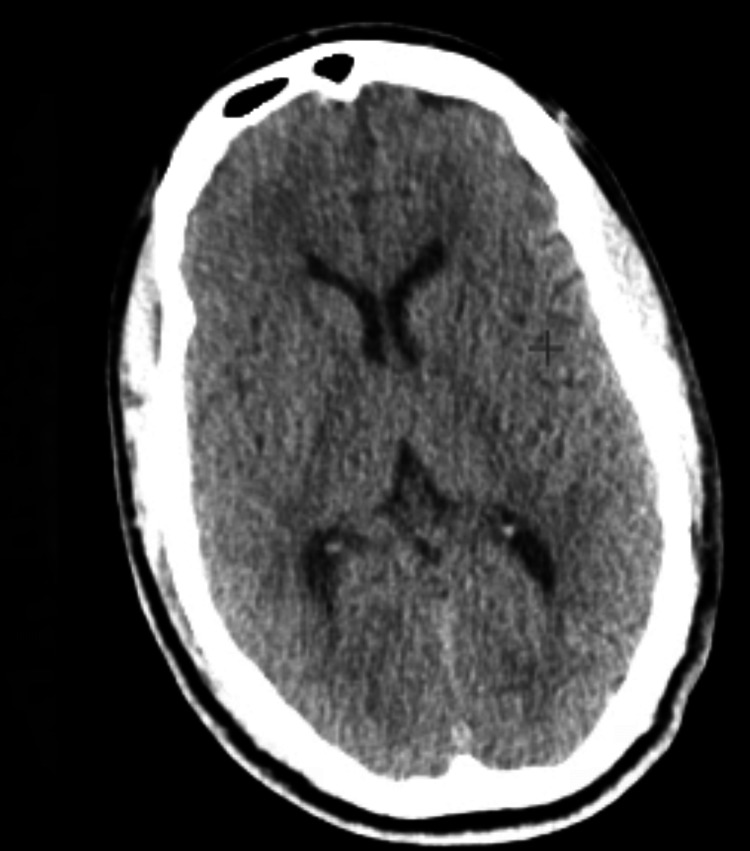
Brain CT from one month following seizure showing normal neuroimaging.
